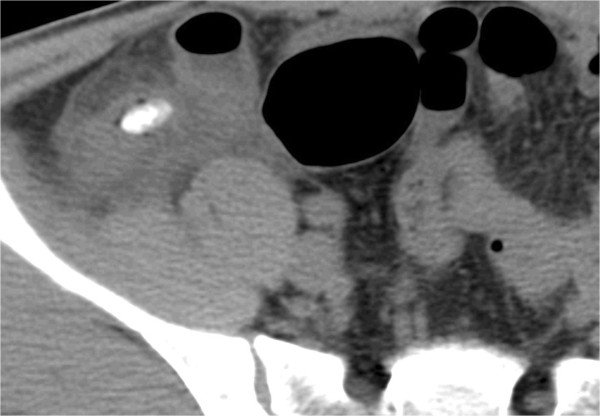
Transverse CT image of 27-year-old man with complicated appendicitis. Appendicolithiasis was observed with involvement of combined fascial plane.
